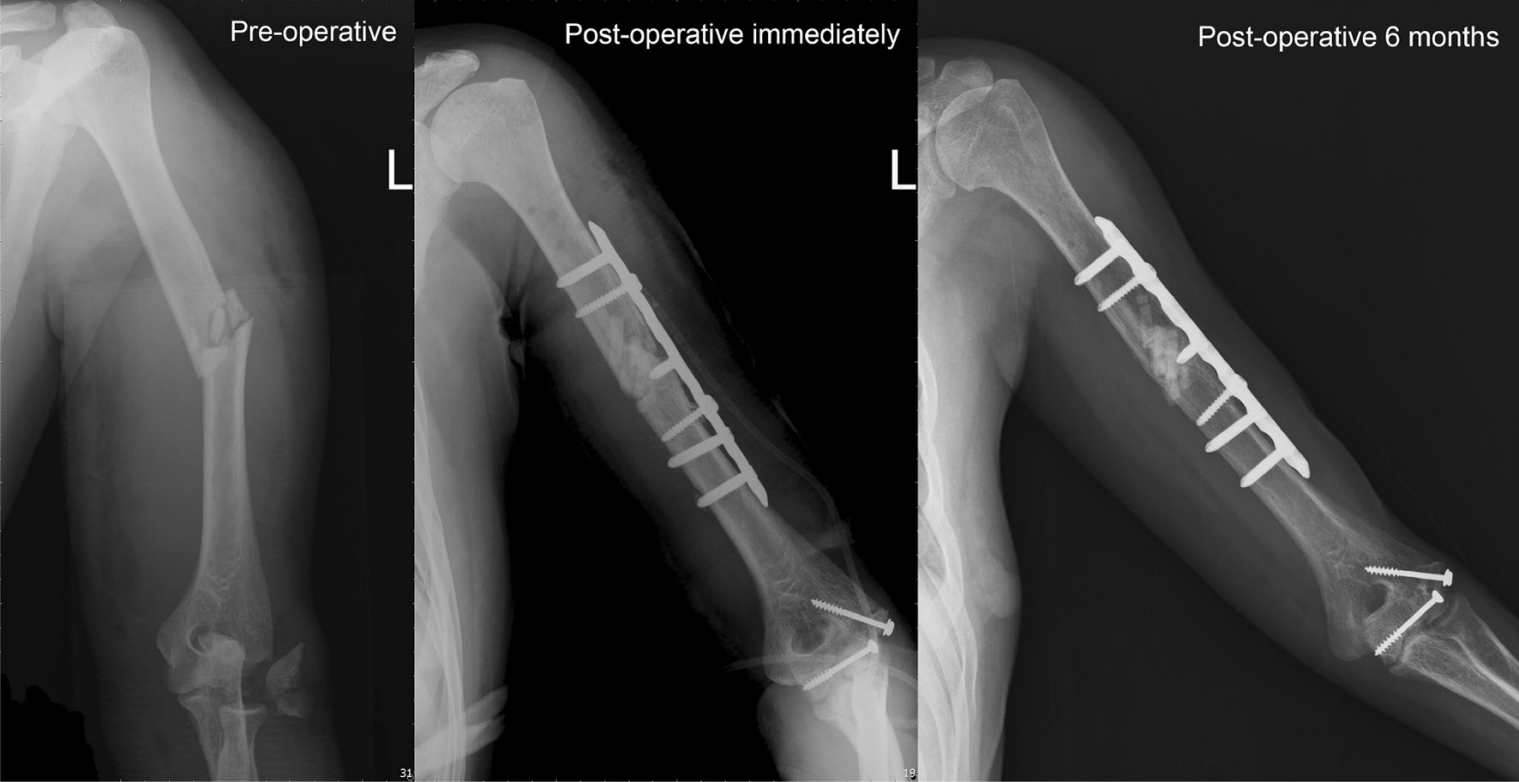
A 40-year-old women had left humeral shaft fracture. She underwent open reduction internal fixation and Bicera™ was filled into fracture (comminuted fracture). Radiograph revealed fracture union 6 months after operation
